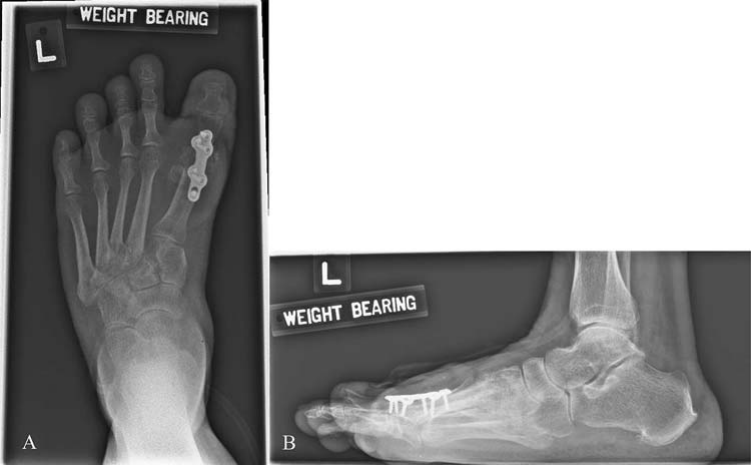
AP (A) and Lateral (B) X-ray the left foot. Metal implants seen over dorsal MTPJ of the 1st toe after corrective hallux valgus surgery.
